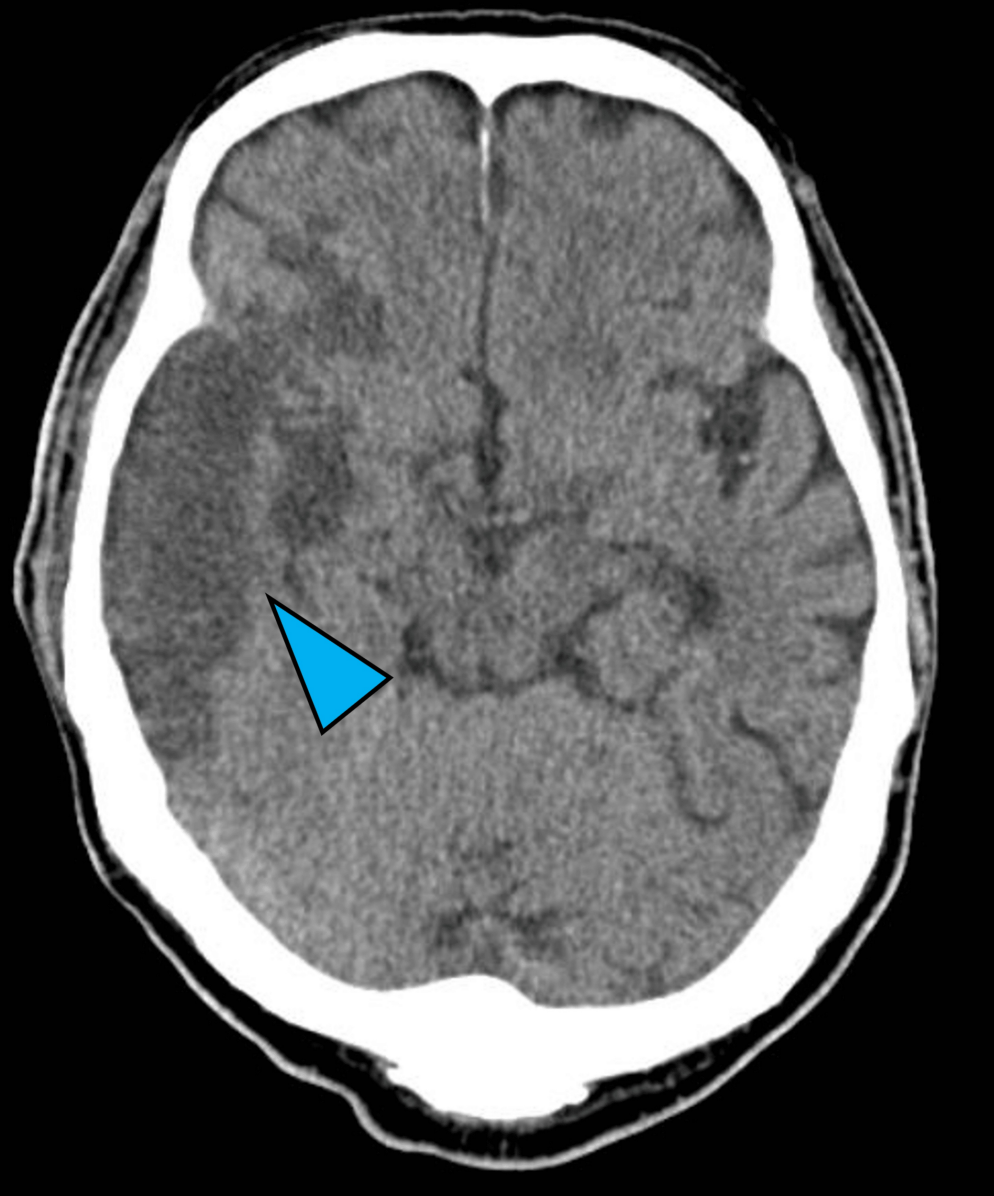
Brain CT on Day 6. Follow-up CT revealed extensive low-density areas in the right MCA region (blue arrowhead). CT: computed tomography, MCA: middle cerebral artery.
